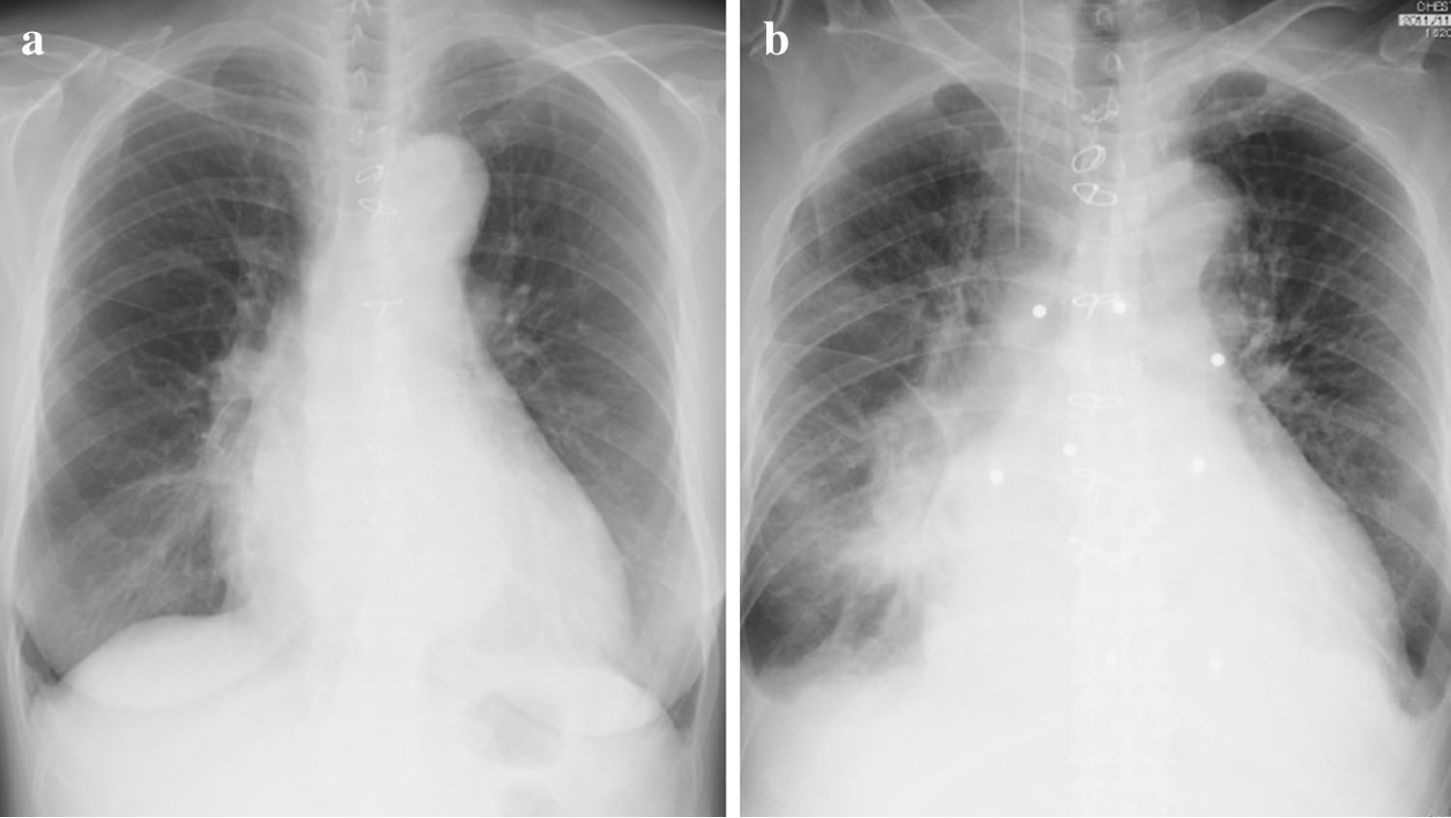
a Chest radiography on admission. b Chest radiography just before surgery
